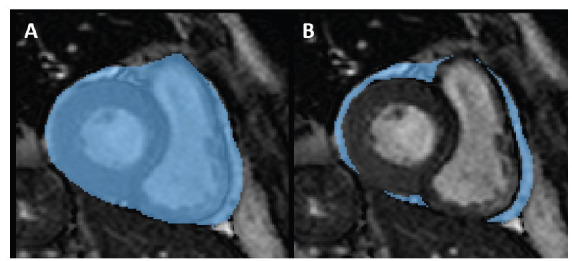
Segmentation of the pericardium (A) with subsequent subtraction of the epicardium (B) to obtain an estimate of epicardial adipose tissue volume in an image obtained from a cine sequence in short axis acquired on an open-bore MRI scanner in a 44-year-old male patient referred for bariatric surgery.
